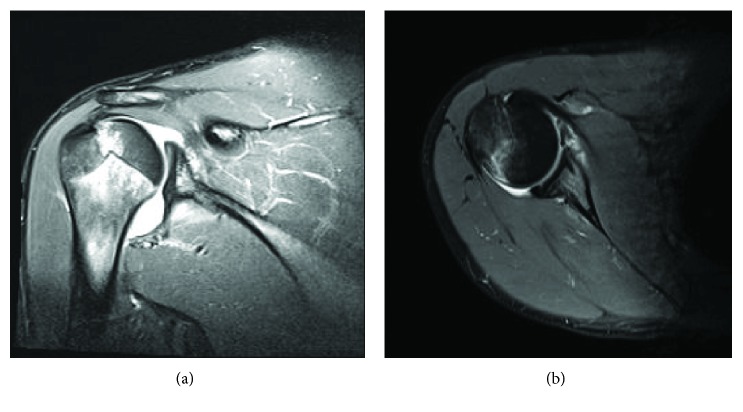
Coronal (a) and axial (b) MRI performed 5 months postoperative due to traumatic injury reveals a Hill-sachs lesion. After nonconservative treatment resulted in improvement, the patient was instructed to follow-up only as needed.
